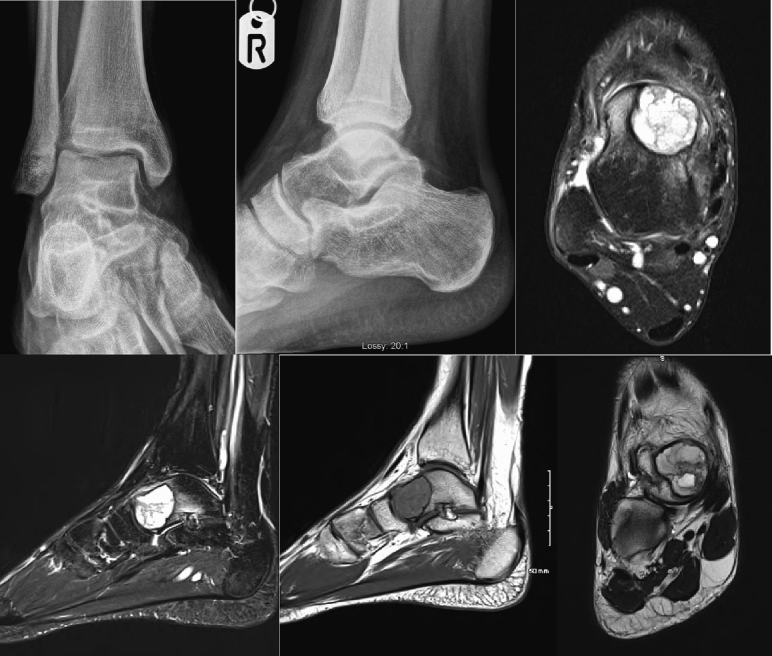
(A, B, C, D, E, F): Pre-operative AP and Lateral Ankle radiograph showed a lucent lesion within the talar neck No periosteal reaction was identified. There was no pathologic fracture. Alignment the ankle mortise was normal. Preoperative MRI demonstrated a 2.5 cm cystic mass in anteromedial aspect of talus with possible slight expansion of the overlying bone. There was extensive surrounding bone oedema in the distal half of the talus, predominantly the neck and subarticular aspect of the talonavicular joint. This was non-homogenous. The ankle joint appeared normal with an appropriate amount of fluid. There was a normal amount of fluid in the small joints of the foot and the ligaments and tendons were all intact.
